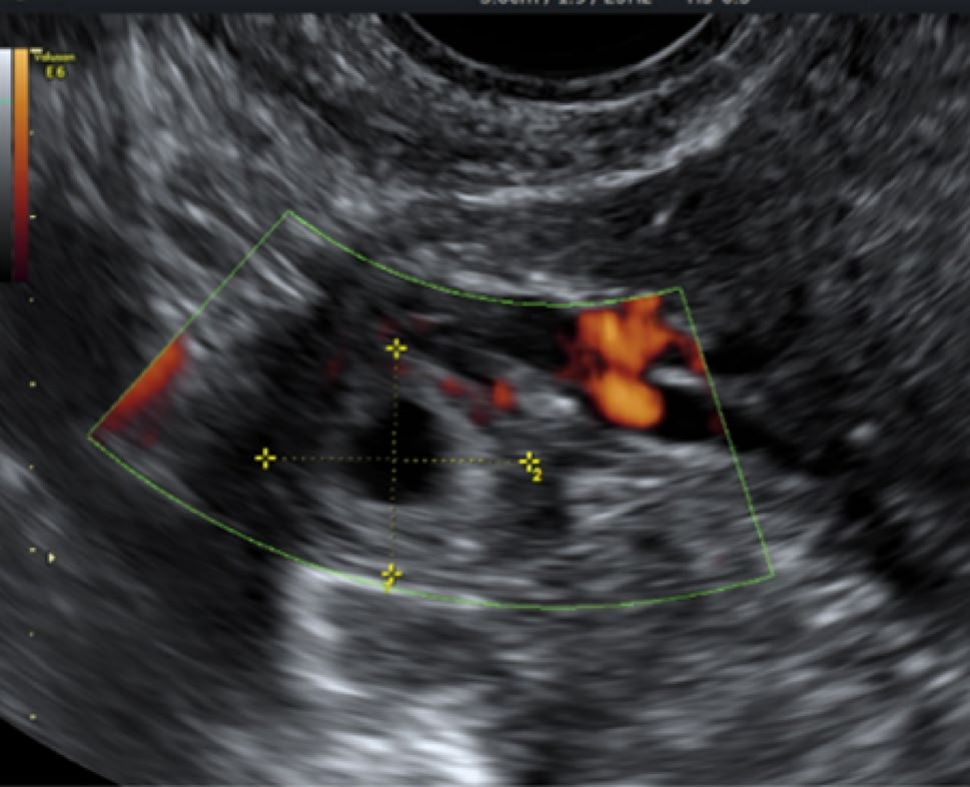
Transverse color Doppler scan showing a hyperechoic tubal ring of an ectopic gestation with no blood flow in the echogenic ring surrounding the gestational sac
